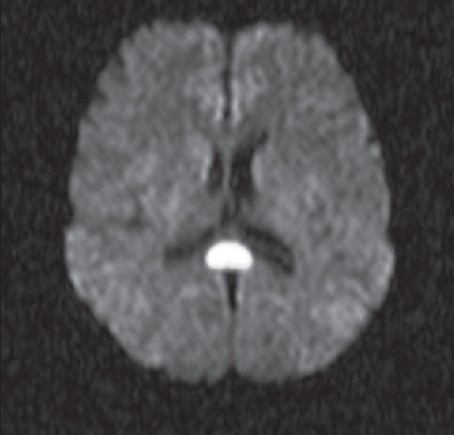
Axial diffusion-weighted MRI sequence shows a well-defined ovoid nodular lesion with restricted diffusion in the center of the splenium of the corpus callosum
